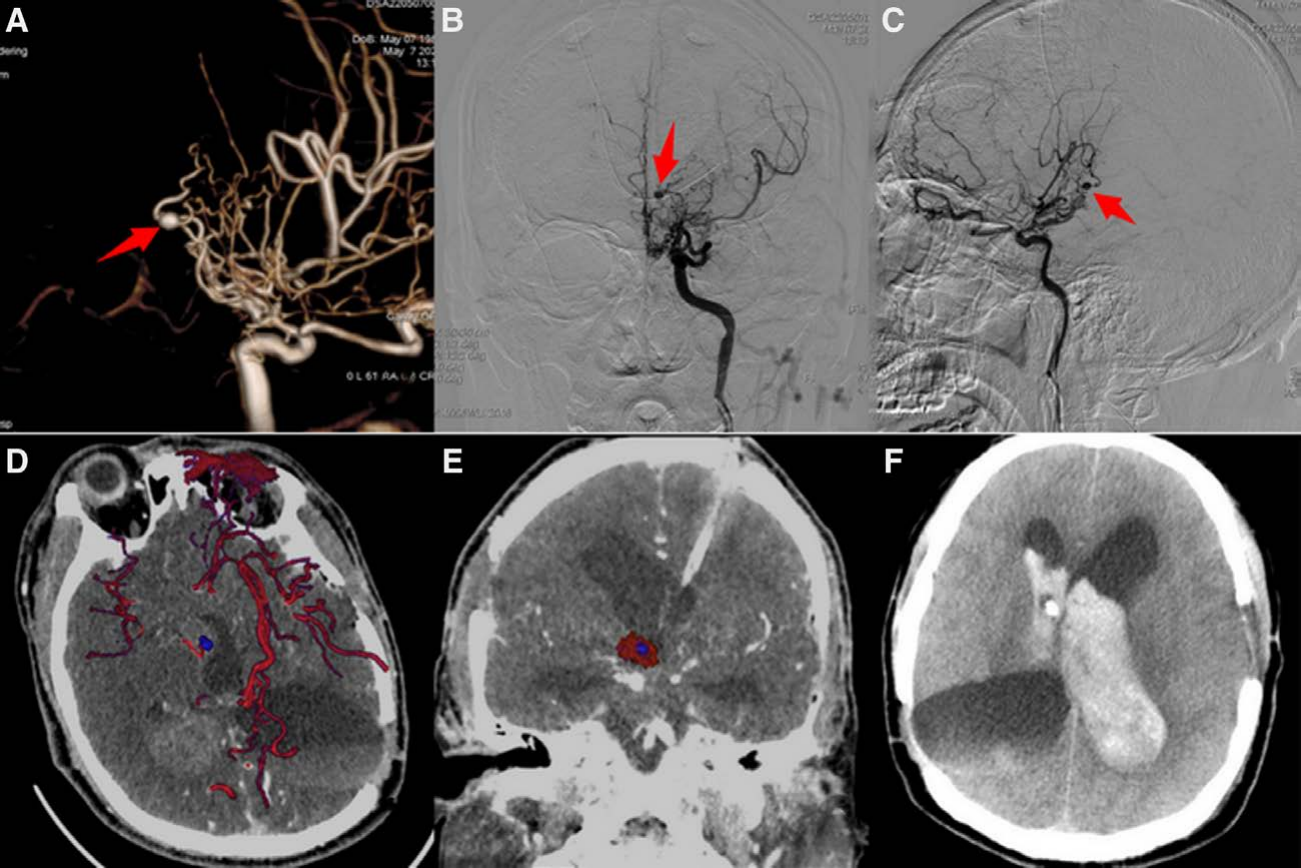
Preoperative imaging results of the patient. (A,B, and C) are images taken during the brain DSA examination while the patient was hospitalized and clearly show the 3-dimensional interaction between the aneurysm and the cerebral arteries (red arrows mark the aneurysm). (D, E) are available images before the operation. Multimodal CTA images clearly show the relationship between aneurysm, ventricle, and hematoma (red partarrows mark the hematoma, blue partarrows mark the aneurysm). (F) is the CT slice showing increased intraventricular hemorrhage before surgery. CT = computerized tomography, CTA = computerized tomography angiography, DSA = digital subtraction angiography.
